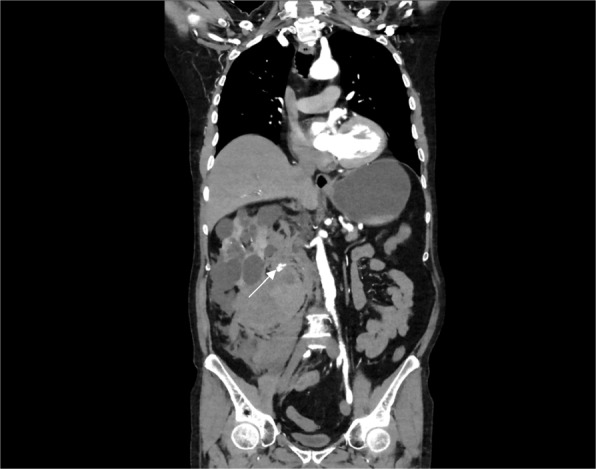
Reformatted Coronal CT scan image demonstrating patency of the main renal artery and area of extravasation involving the lower pole region of the polycystic kidney (denoted by a white arrow)
